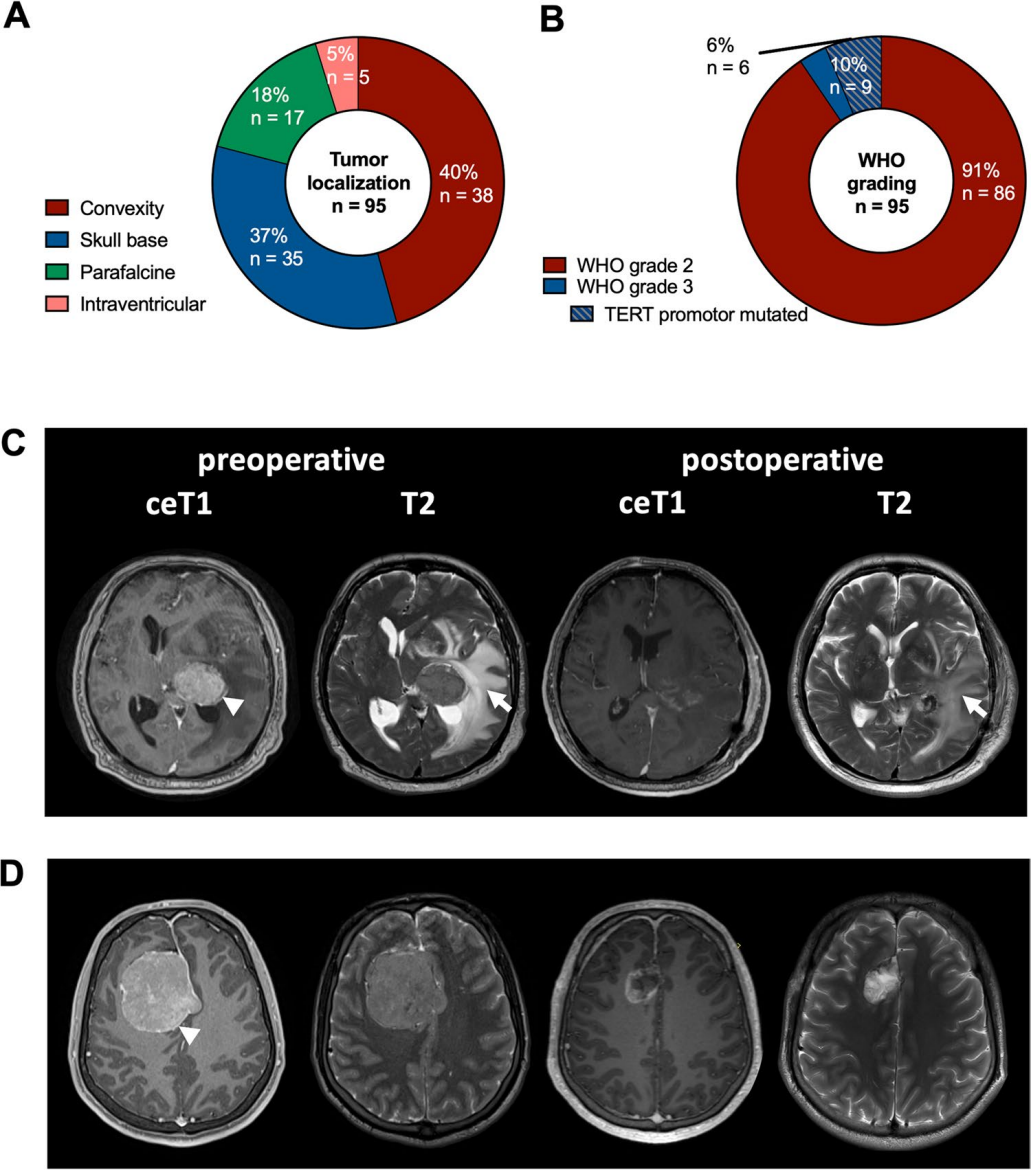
Tumor characteristics and case examples. A Distribution of tumor localization across the entire study cohort (*n* = 95). B Distribution of tumor grading according to the 2021 WHO classification including TERT promotor mutation status in the entire study cohort (*n* = 95). C Preoperative and postoperative axial contrast-enhancing T1-weighted MRI demonstrating homogenous contrast enhancement of a left-sided intraventricular meningioma WHO grade 2 (arrowhead) with no apparent residual tumor after resection. T2-weighted MRI sequences showing extensive preoperative perifocal edema slowly decreasing on postoperative imaging. D Preoperative axial contrast-enhancing T1-weighted MRI demonstrating homogenous contrast-enhancement of a right-hemispheric frontal parafalcine meningioma WHO grade 2 (arrowhead) without perifocal edema on T2-weighted MRI. Postoperative MRI showing complete tumor resection
